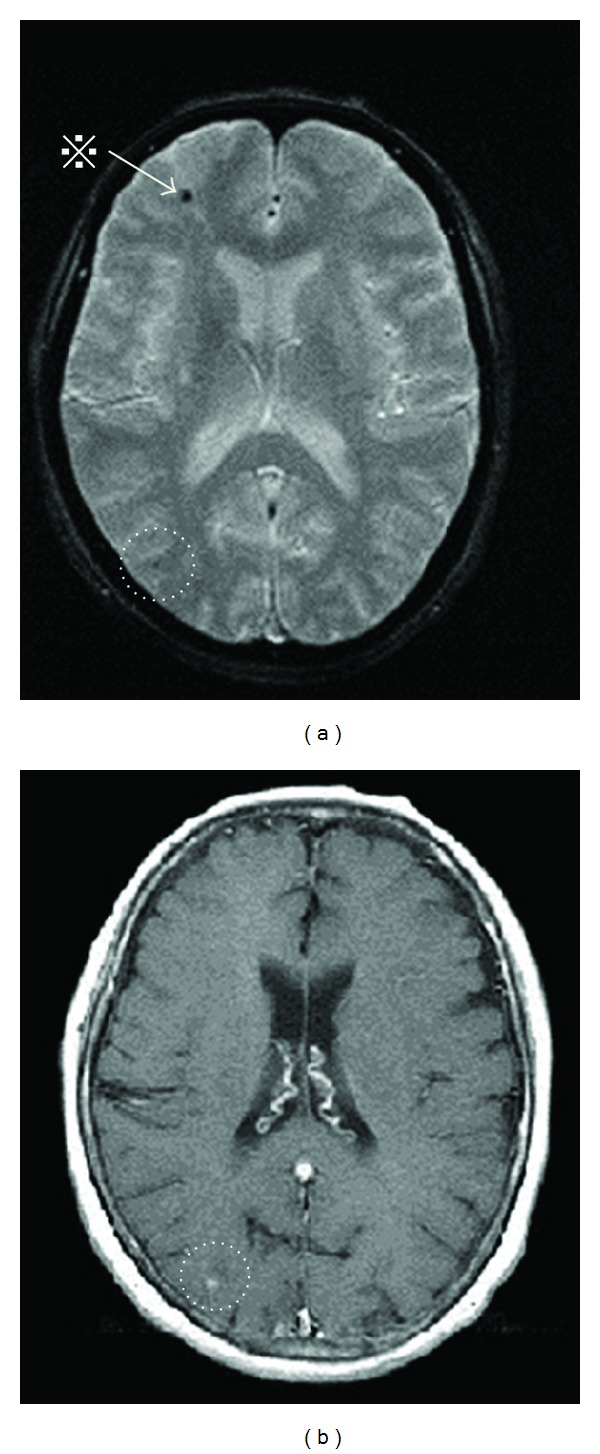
Black dots on T2* weighted brain magnetic resonance imaging (a) and the gadolinium (Gd) contrast medium enhancement effect. (b) *※* a black dot on the right frontal lobe does not show the enhancement effect seen at (b) (i.e., no fresh microbleeding).
